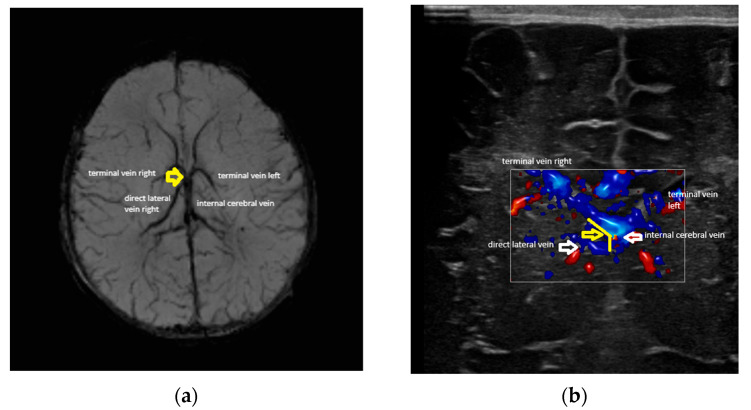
(a) MRI of deep brain venous system. (b) Colour Doppler (same patient).
